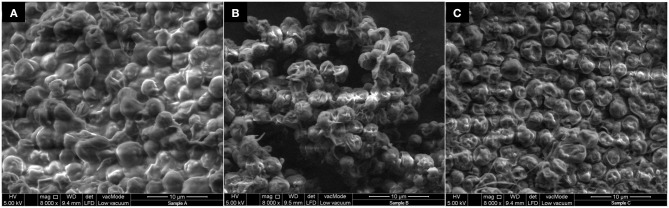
FESEM images of microalgae cells (A) before sonication treatment; (B) after ultrasound with water as medium; (C) after ultrasound with UATPP medium.
